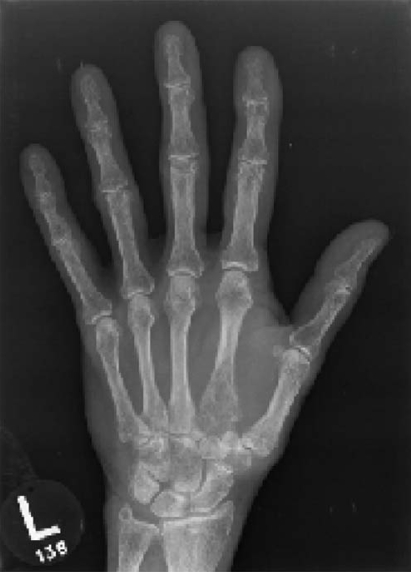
Metastatic bronchogenic carcinoma in the second metacarpal base. Radiograph of the left hand reveals a large, osteolytic, permeative, sightly expansile lesion in the base of the second metacarpal extending from the subchondral bone to the metacarpal shaft, with associated soft-tissue extension.
